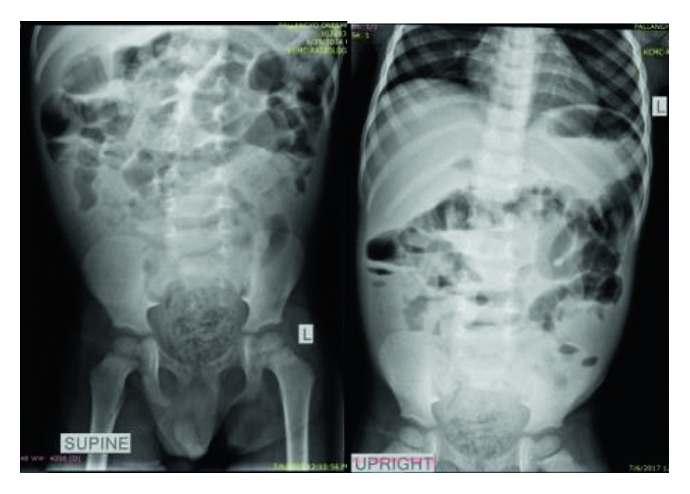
Plain abdominal X-rays (erect and supine) showing proximal small bowel loop dilatation, gaseous with some air fluid levels, while the distal part being dense with the rectum loaded with fecal matters.
